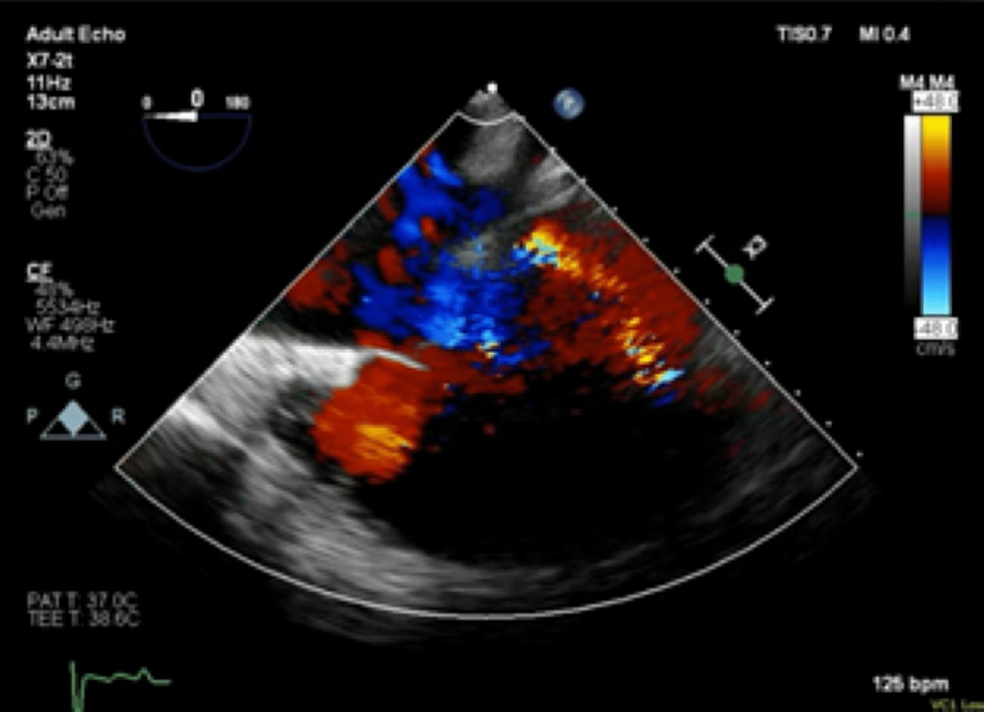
Transesophageal echocardiogram confirming the findings of biatrial cardiac shunting by the previous two TTE studies TTE: Transthoracic echocardiogram
